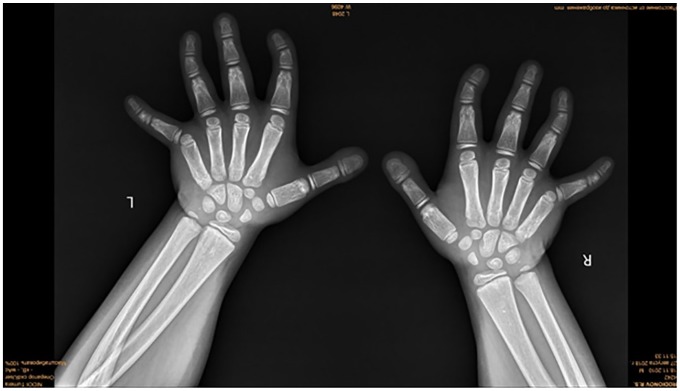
Neither Madelung’s deformity nor radioulnar dislocation. Anteroposterior radiograph of the forearms in a 5-year-old boy with Robinow syndrome, autosomal dominant type, showed normal development of the radioulnar with normal pronation and supination. No camptodactyly, though rectangular first metacarpals, multiple pseudoepiphyses, and clinodactyly, were noted.
